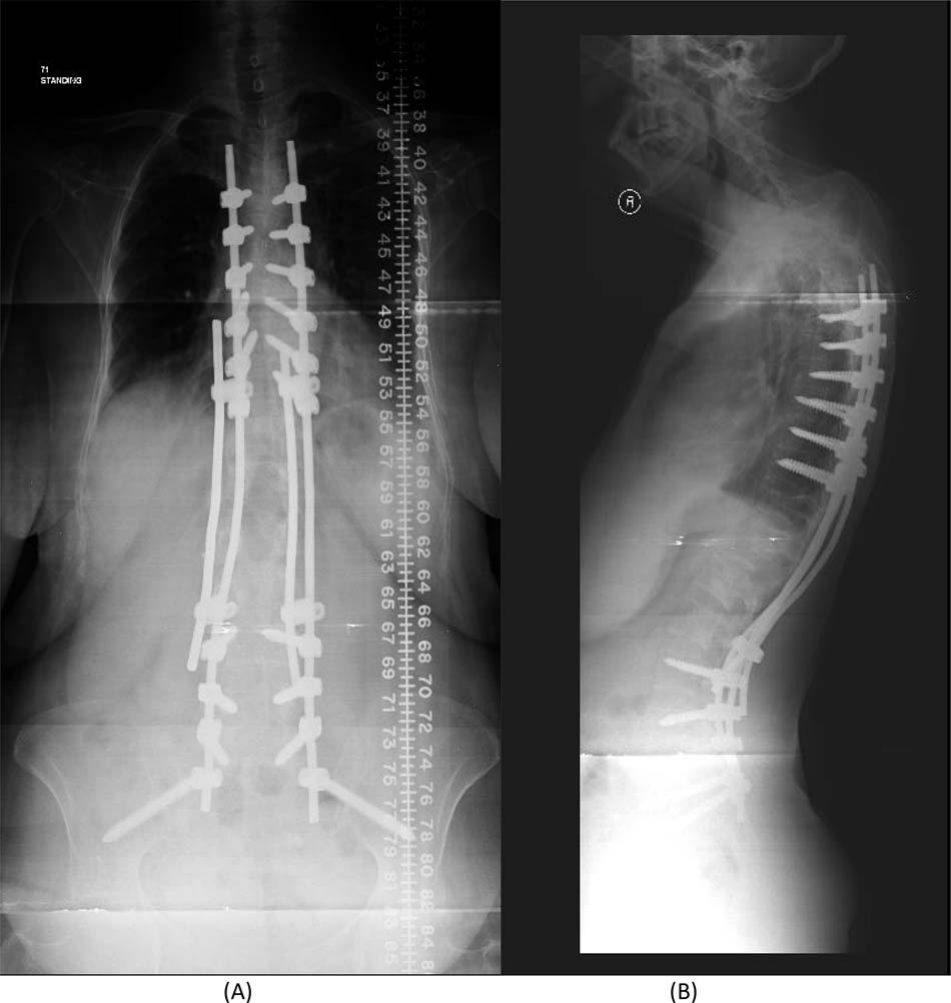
(A) AP lumbar spine X-ray demonstrating the bridging technique. (B) Lateral spine X-ray showing the bridging technique sparing the infected area.
